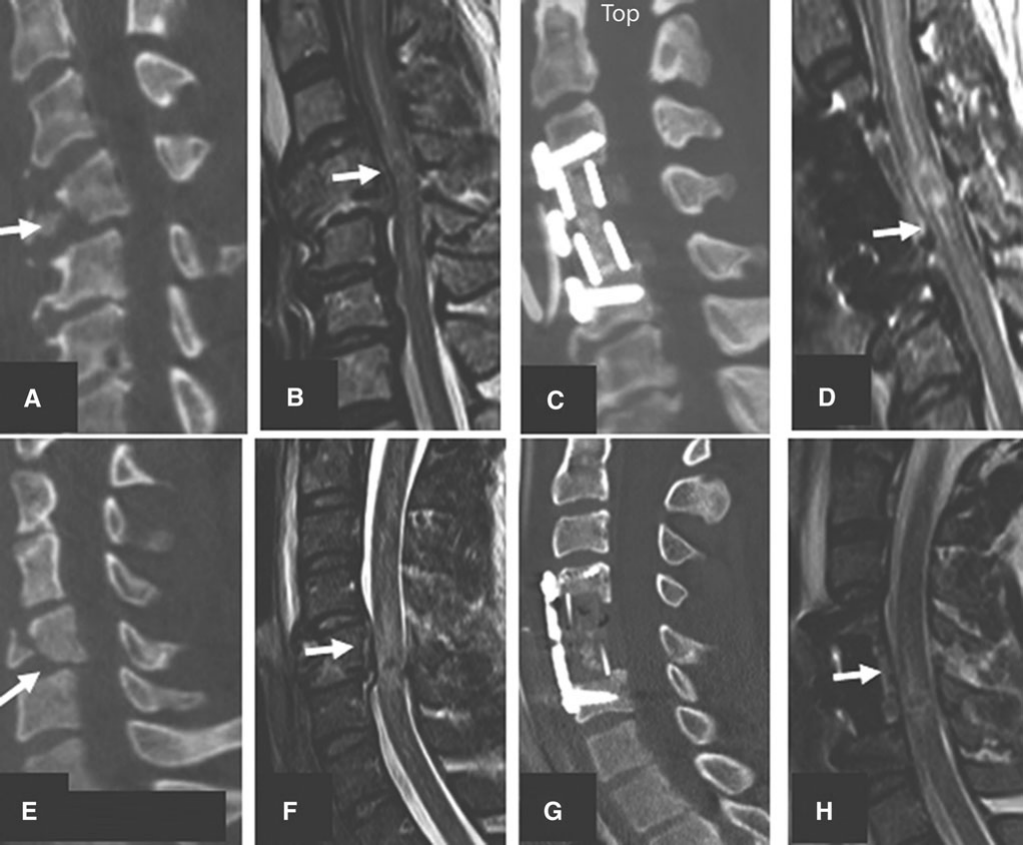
Midsagittal CT and MRI of one level ACCF with and without success in decompression. (A-D) indicate a teardrop fracture (A and B arrows) indicating compressed spinal cord at C4 and beyond. (C) and (D) show one level corpectomy with no evidence of decompression (D). (E) and (F) indicate a C5 tear drop fracture managed with corpectomy and successful decompression of spinal cord (G and H arrows).
